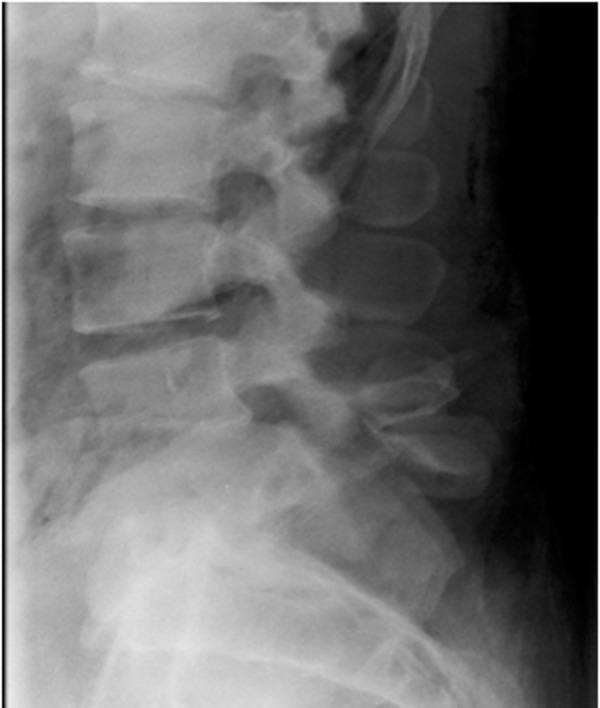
Preoperative lateral x-ray This is a preoperative lateral x-ray demonstrating a fracture dislocation with a posterior retrolisthesis of the L5 vertebrae over S1.
